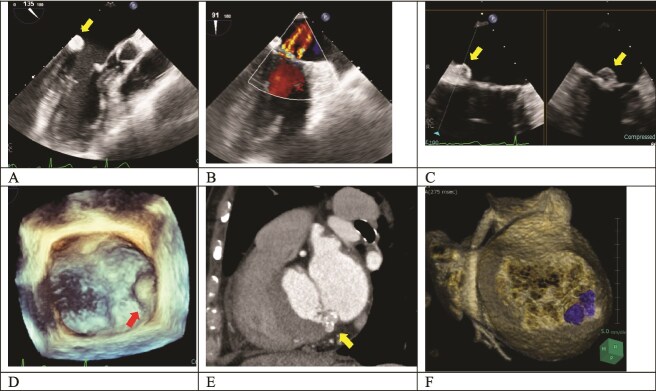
Preoperative images. (A) Echocardiography showing a hyperechoic mass (arrow) at the posterior leaflet of the mitral valve. (B) Color Doppler echocardiography demonstrating mild mitral regurgitation. (C) A mass lesion (arrows) with an internal hypoechoic core is observed attached to the posterior mitral annulus, accompanied by acoustic shadowing. (D) A protruding lesion (arrow) was observed within the left atrium at the P3 segment of the mitral valve on the reconstructed image. (E) CT showing a calcified mass (arrow) with an internal low-attenuation area. (F) Three-dimensional reconstruction image revealing a mass lesion occupying the posterior mitral annulus (P3 segment).
